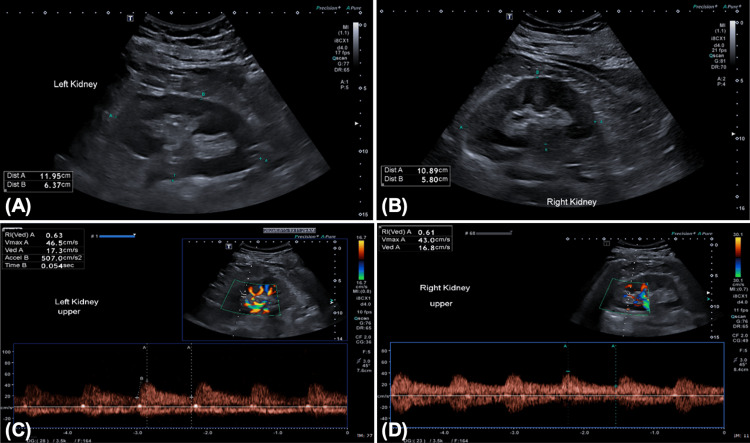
Grey-scale (A and B) and colour Doppler (C and D) ultrasound images showing bilateral normal-sized kidneys with normal echogenicity, cortical thickness, and duplex parameters.
